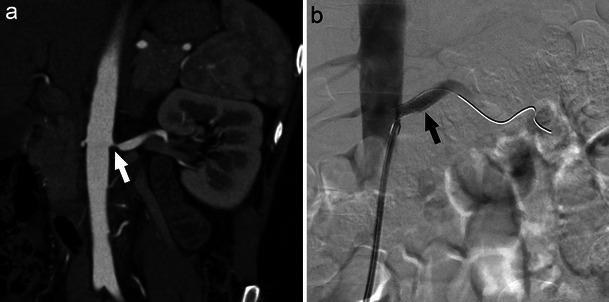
CT angiography demonstrating significant left proximal main renal artery stenosis (a, white arrow) managed with percutaneous transluminal renal angioplasty and stent placement (b, black arrow)
